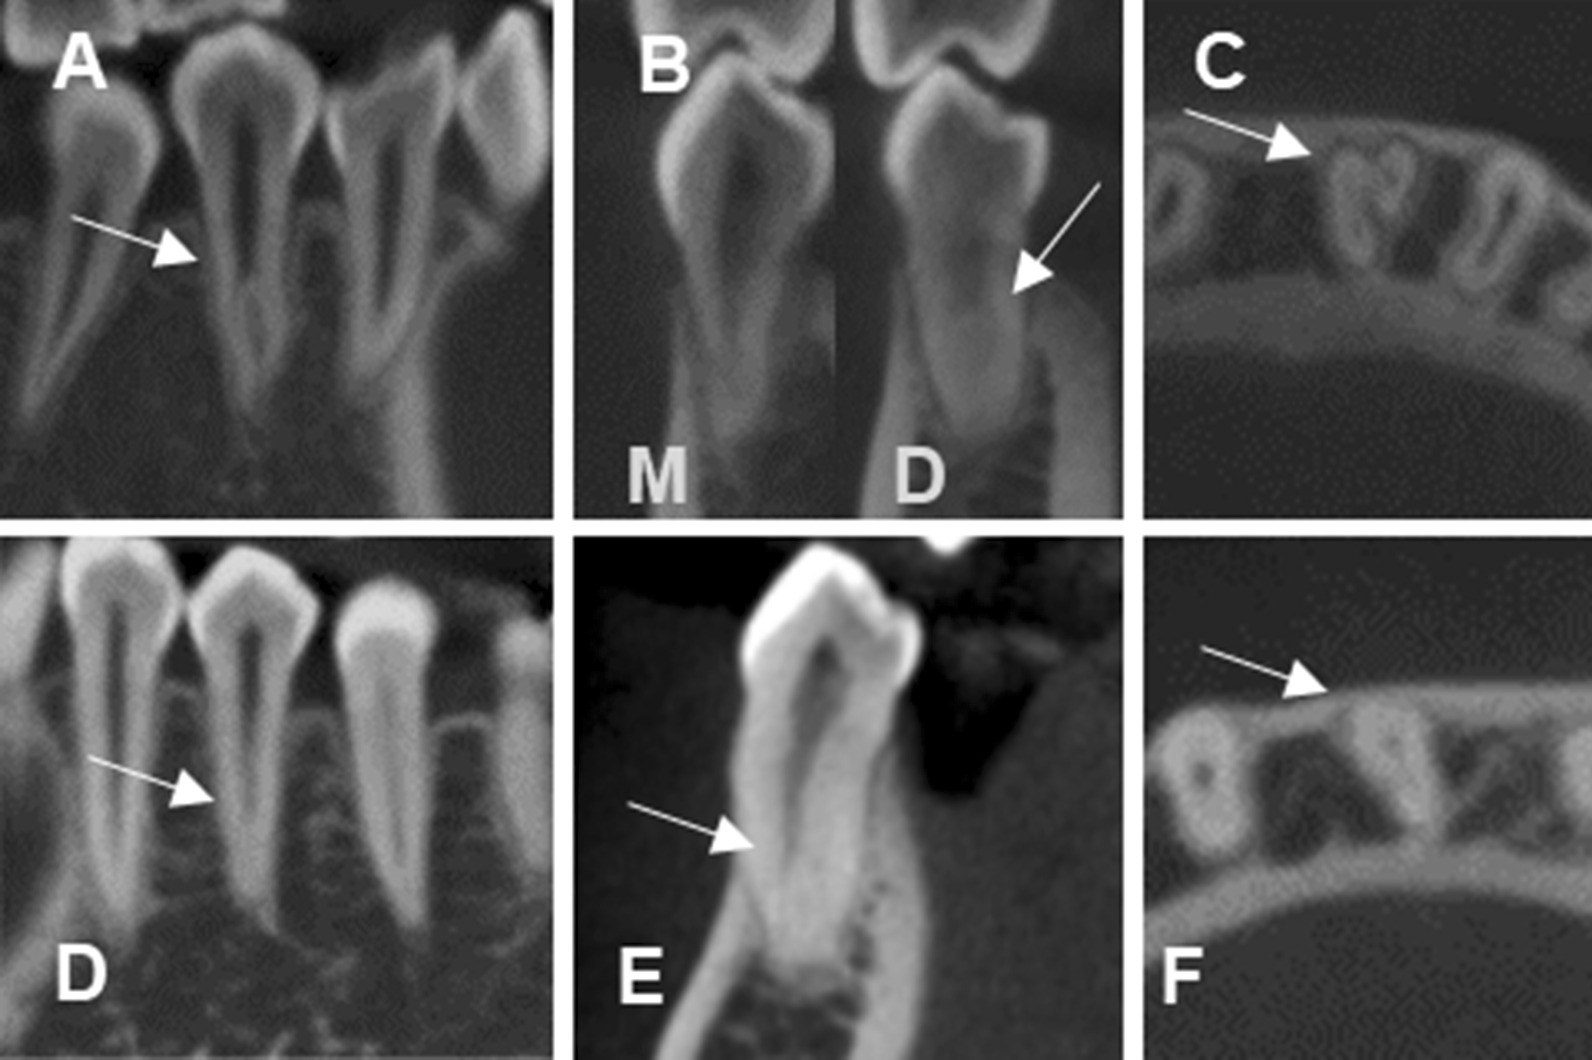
A–C CBCT images of a mandibular first premolar with three root canals. A Sagittal view of a 2-rooted mandibular first premolar with mesial and distal roots. B Coronal views of a mesial root with 1 root canal and distal root with 1 root canal extending from the pulp chamber and dividing into 2 root canals at the middle 1/3 level. M indicates mesial root and D indicates distal root. C Axial view of mesial, distobuccal, and distolingual root canals. D–F CBCT images of a mandibular first premolar with two root canals. D Sagittal view of a single-rooted mandibular first premolar whose root canal initiates at the CEJ and disappears in the apical third level (fast break). E Coronal view of 1 root canal extending from the pulp chamber and dividing into 2 root canals at the middle 1/3 level. F Axial view of 2 root canals at the middle 1/3 level. Arrows indicate mandibular first premolars
